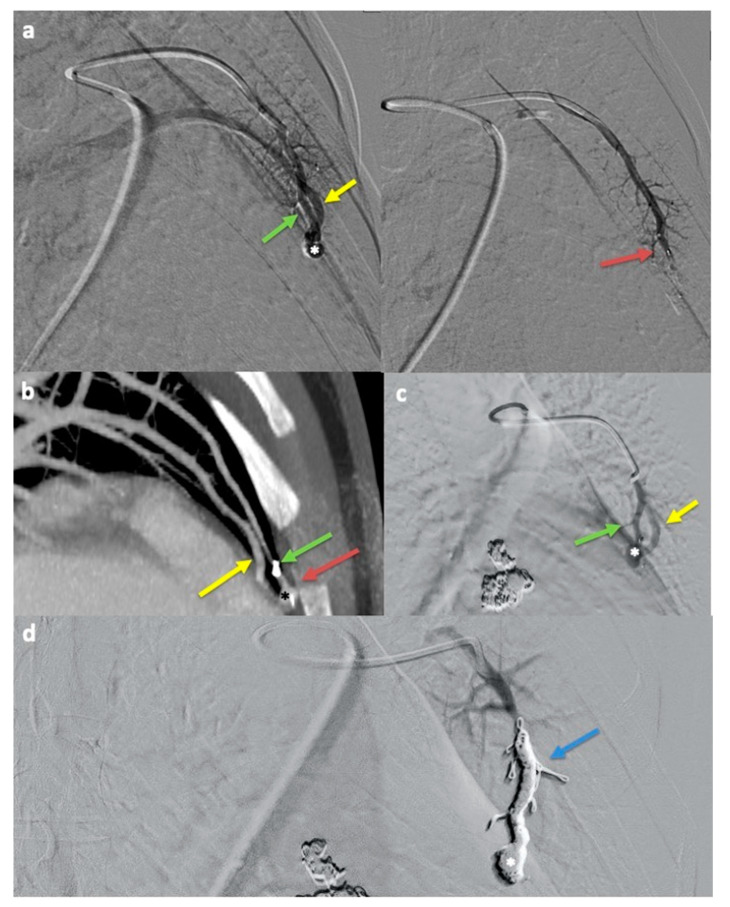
Treatment failure and retreatment after feeding artery (FA) microvascular plug (MVP) embolization. (a) Digital subtraction angiography before (left) and after (right) feeding artery (FA) (green arrow) microvascular plug (MVP) (red arrow) embolization of a pulmonary arteriovenous malformation (PAVM) in the lingula. The draining vein (yellow arrow) is seen opacified before but not after embolization. (b) Coronal maximum intensity projection (MIP) CT angiography obtained 14 months after the procedure demonstrates residual opacification in the nidus (asterisk) and draining vein (yellow arrow) distal to the MVP (red arrow) and FA (green arrow), consistent with treatment failure due to recanalization. (c) Digital subtraction angiography 5 years after the initial procedure demonstrates recanalization of the nidus (asterisk) and draining vein (yellow arrow) distal to the FA (green arrow). (d) Following coil embolization of the dominant feeding artery and nidus using a Ruby 6 mm × 30 cm soft coil and two 60 cm packing coils (blue arrow), the draining vein and nidus (asterisk) no longer opacify.
